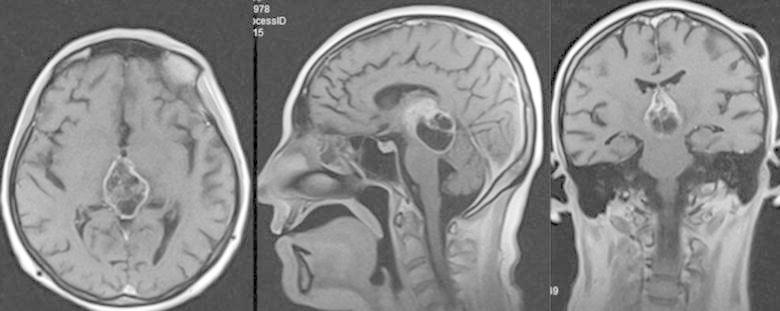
Post-operative 6th month brain MRI depicts resolution of hydrocephalus and more necrotic consistency of the lesion
